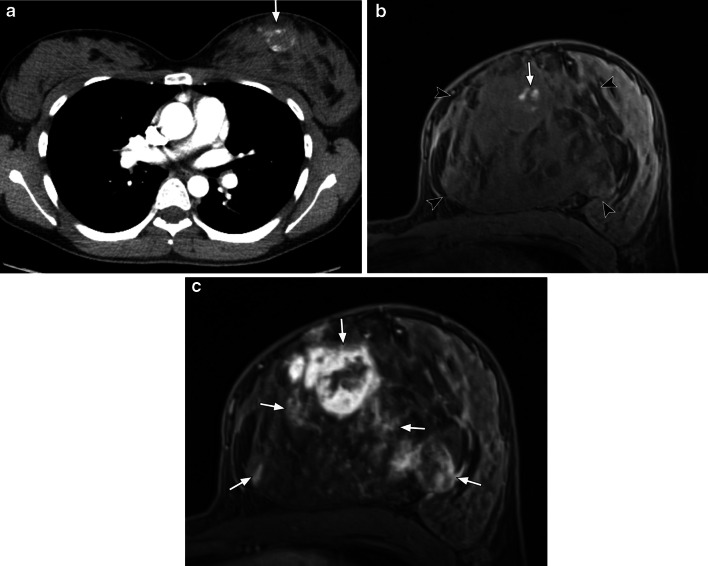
27-year-old woman with primary left breast angiosarcoma. a Contrast-enhanced axial CT image shows a lesion in the medial left breast with peripheral rim enhancement (arrow). b Axial T1-weighted fat-suppressed pre-contrast image shows a large mass (arrowheads) occupying much of the left medial and central left breast which displaces the normal fibroglandular tissues laterally. There is a small area of high T1 signal within the mass suggestive of haemorrhage (arrow). c Axial T1-weighted fat-suppressed early dynamic post-contrast image shows multifocal areas of variable heterogeneous peripheral enhancement (arrows). The extent of disease was underestimated on CT
